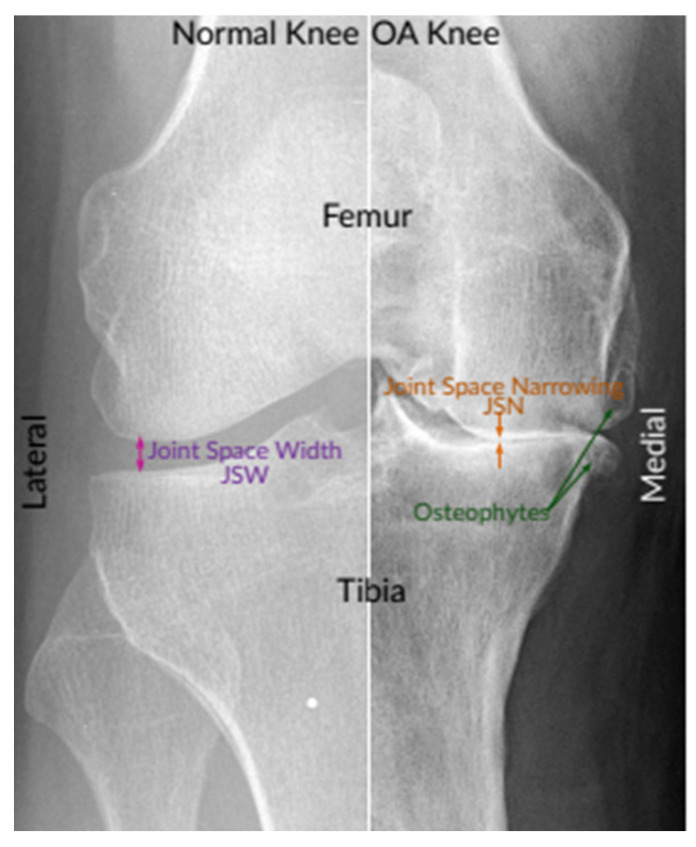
An instance of X-ray images of the normal knee and severe OA knee. On the left is a normal knee, and on the right is an OA knee. This image shows joint compartments, as well as joint space narrowing (JSN) and osteophytes [10].
